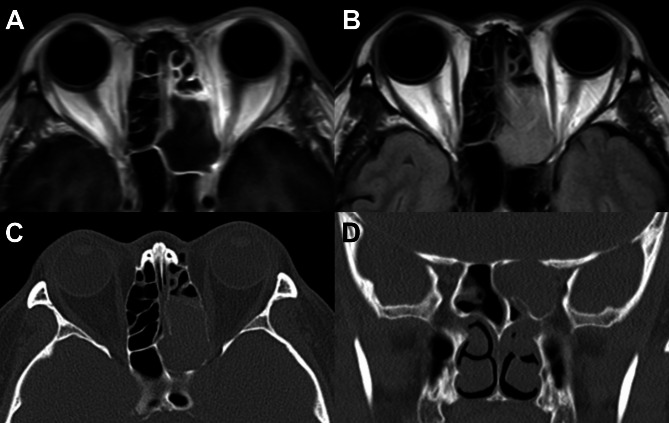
Preoperative Brain Magnetic Resonance Imaging (MRI) and Paranasal Sinus Computed Tomography (CT). (A, B) Preoperative brain MRI shows mass on the ethmoidal sinus, with intermediate signal on T1 image (A) and intermediate to low signal intensity on T2 FLAIR image (B). (C, D) Preoperative paranasal sinus CT. (C) Axial scan shows total opacification on the left ethmoidal cell. (D) Coronal scan shows prominent dehiscence of canal wall (arrowheads)
